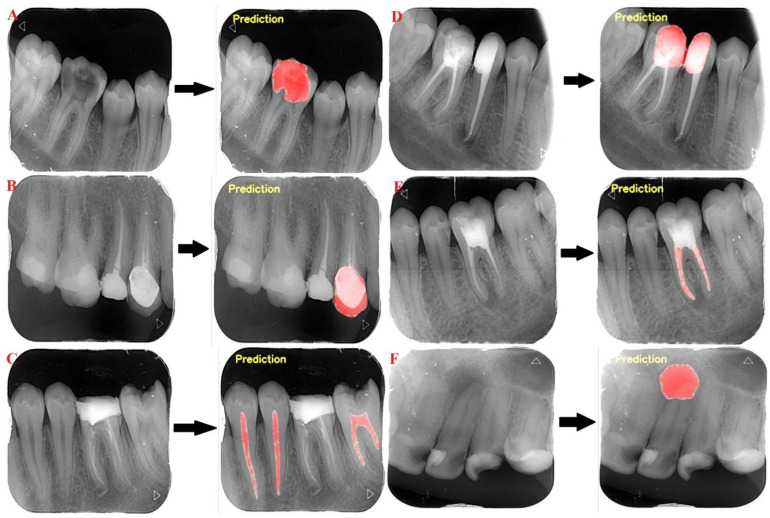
Dental conditions’ segmentation on periapical radiographs using the AI model ((A) caries, (B) crown, (C) dental pulp, (D) filling, (E) root canal filling, (F) periapical lesion).
